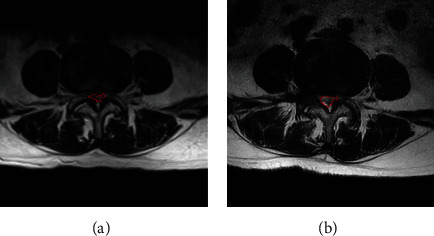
The cross-sectional area of the dural sac at the maximal compression level of a case with supine MRI scans. (a) Preoperative and (b) postoperative T2-weighted images plotted and measured on axial view using PACS. PACS = Picture Archiving and Communication System.
